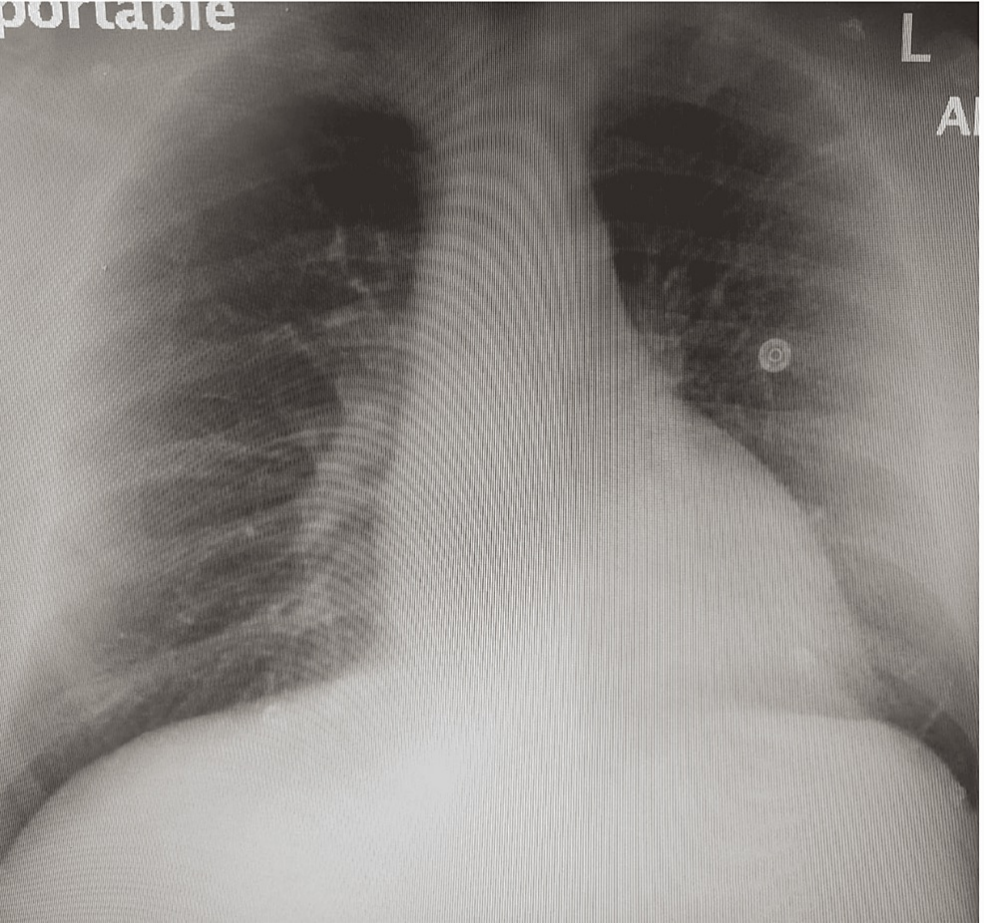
Normal portable chest x-ray.
